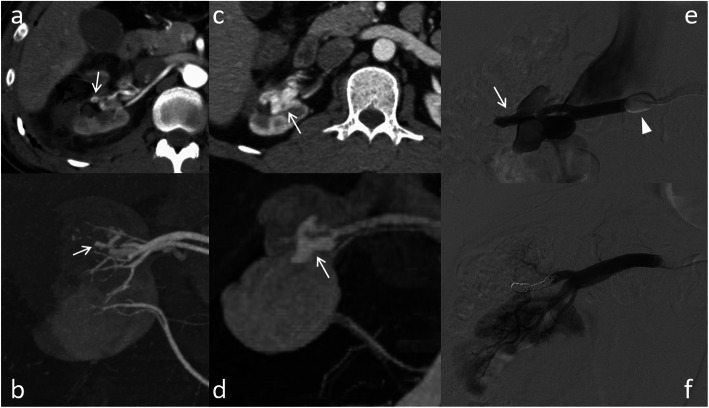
A case of an increasing arteriovenous fistula (AVF), without symptoms, in a 40-year-old man. a, b Irregularly shaped enhancement (arrows) is suspected on early postoperative contrast-enhanced CT on postoperative day (POD) four. c, d It is increased in size and AVF is suspected (arrows) on follow-up CT after 1 year without symptoms. e The AVF (arrow) is embolized using microcoils under balloon occlusion (arrowhead) on POD 414
